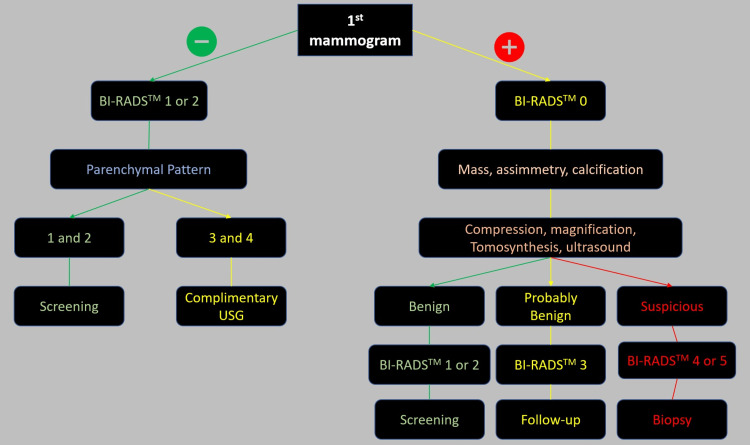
Workflow for the first mammography We have only BI-RADS® 0, 1, and 2 at the first assessment. After complimentary studies, probably benign findings should be considered for benign features without evolutionary control. BI-RADS®: Breast Imaging and Reporting Data System, USG: ultrasonography
